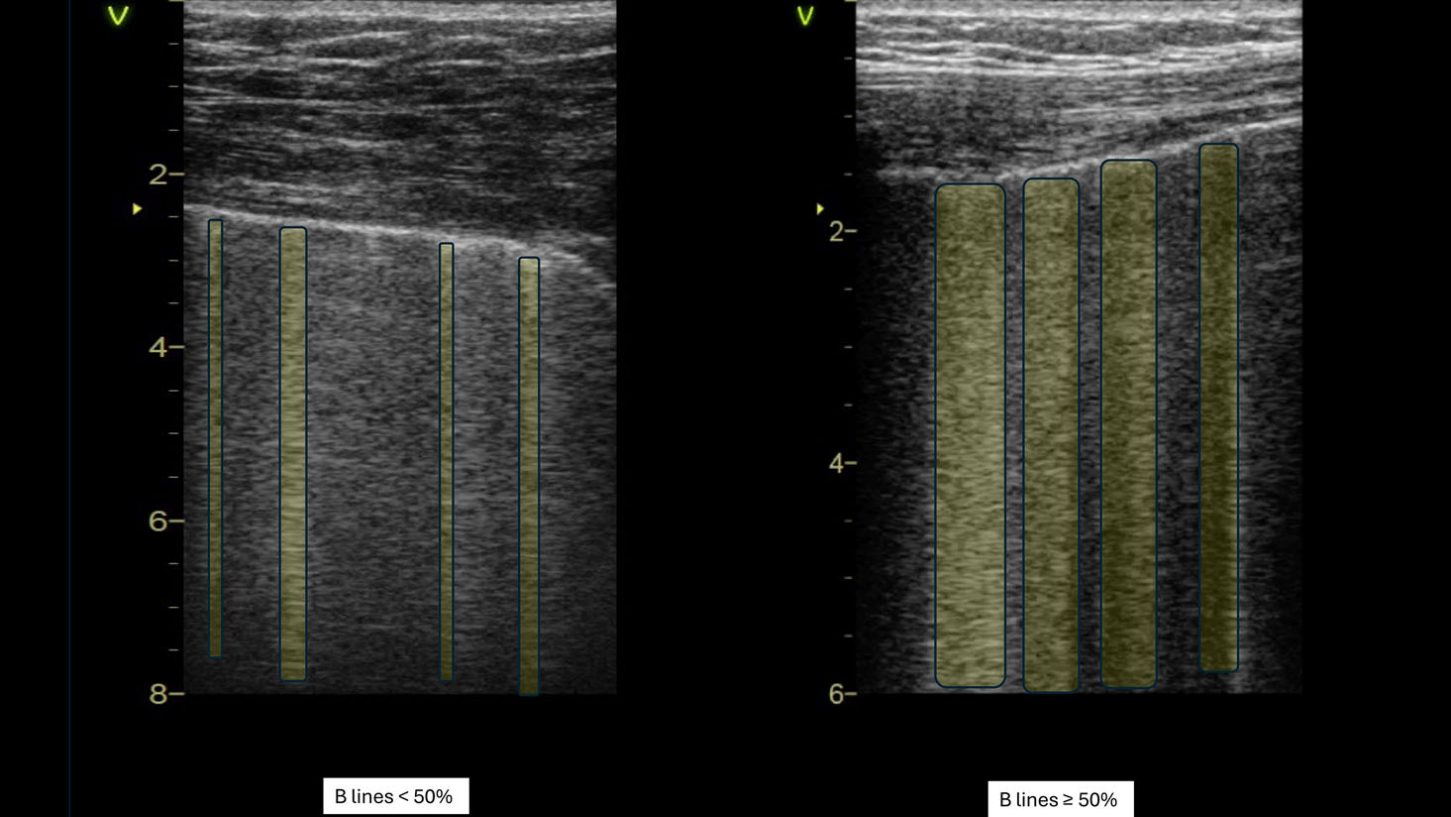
Lung ultrasound dichotomous evaluation. Example of B‐lines occupying <50% of the lung field (*left*) and B‐lines occupying ≥50% of the lung field.
